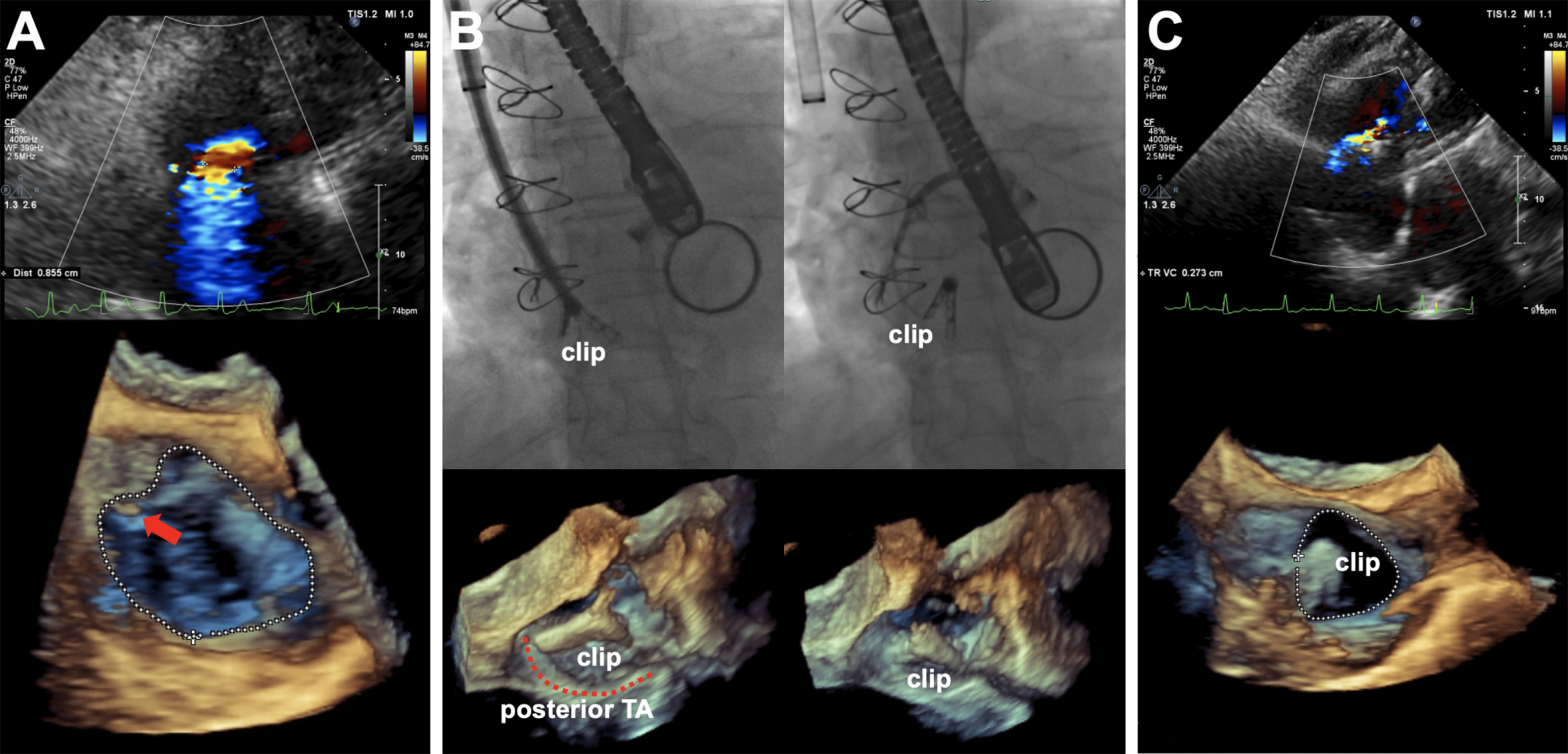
(A) Intraprocedural TEE showing torrential TR with EROA of 0.74 cm^2^, regurgitation volume of 51 ml, and vena contracta width of 0.71 cm (upper panel) and 3D work plane revealing the original knot of the Kay’s procedure (red arrow) and TA morphology before K-Clip™ implantation (lower panel). (B) Real-time fluoroscopy (upper panel) and 3D work plane of TEE (lower panel) showing the opening clamp and the clip on the target location after release. (C) Postprocedural TEE showing an immediate reduction of TR grade with an EROA of 0.16 cm^2^, regurgitation volume of 12 ml, and vena contracta width of 0.27 cm (upper panel) and 3D work plane (lower panel) showing the TA morphology after K-Clip™ implantation (red arrow). TEE, transesophageal echocardiogram; TR, tricuspid regurgitation; EROA, effective regurgitant orifice area; TA, tricuspid annulus.
